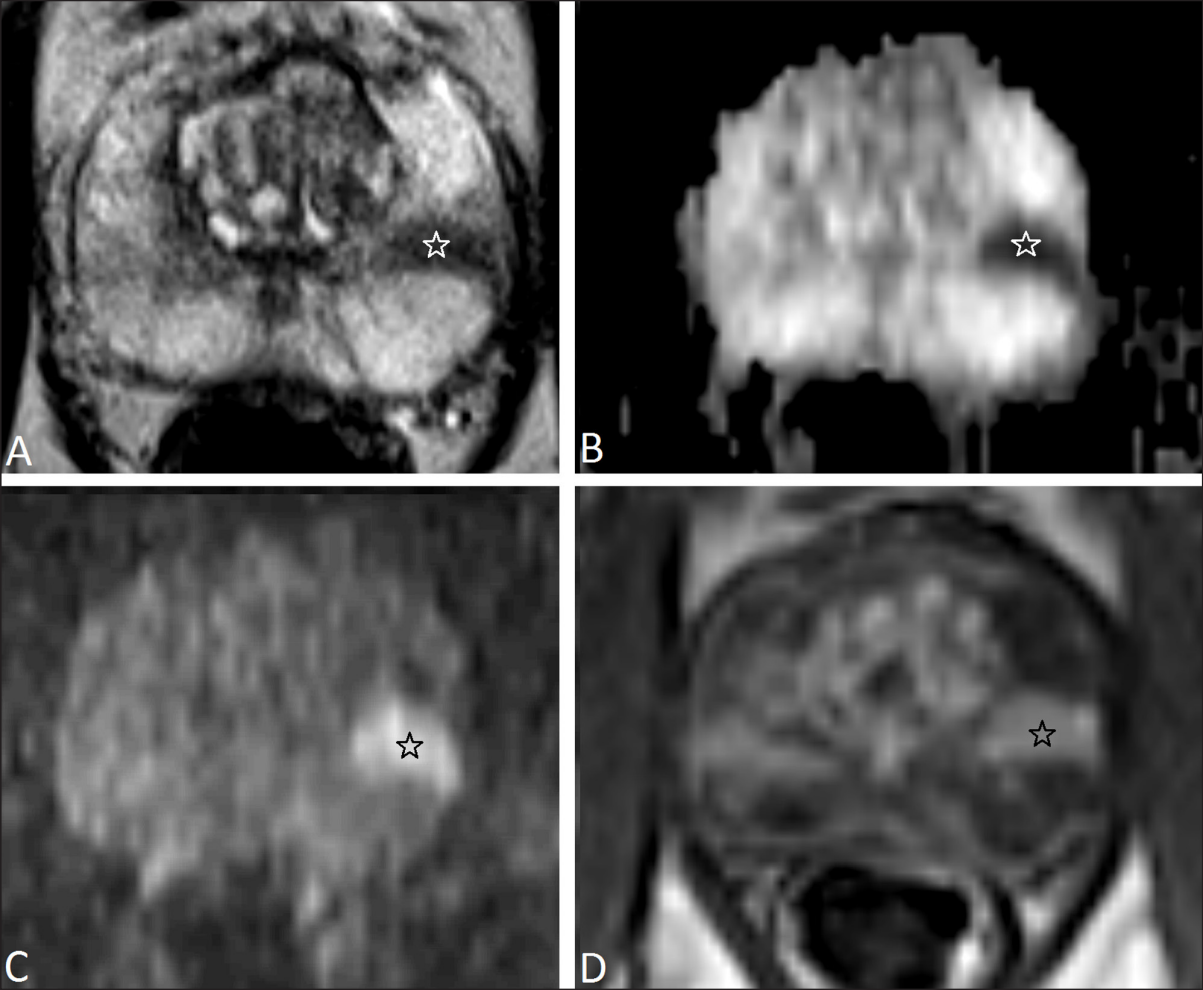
A 63-year-old man with PSA 8.35 μg/L. In the PZ on the left side there is a low signal intensity focus on T2-WI (PI-RADSv1 score 5; PI-RADSv2 score 4) (a) (white star) with restricted diffusion (markedly hypointense on ADC (b) and hyperintense on high-b-value (c)) (PI-RADSv1 score 5, PI-RADSv2 score 4). The size of the lesion is <1,5 cm, it has no broad contact with the prostate capsule and shows no definite extraprostatic extension. On DCE (d) it shows strong contrast enhancement (black star) (PI-RADSv1 score 5; PI-RADSv2 positive). The overall assessment score is 5 when using PI-RADSv1 based on a subjective impression of the findings of all modalities and is 4 in PI-RADSv2 based on the DWI-only which should be given a score 4. Despite the different overall assessment category between PI-RADSv1 and PI-RADSv2 the message to the clinician is the same, i.e. targeted prostate biopsy is warranted. Prostate biopsy followed by radical prostatectomy confirmed a PC with Gleason score 3+4 = 7 (with tertiary pattern 5).
